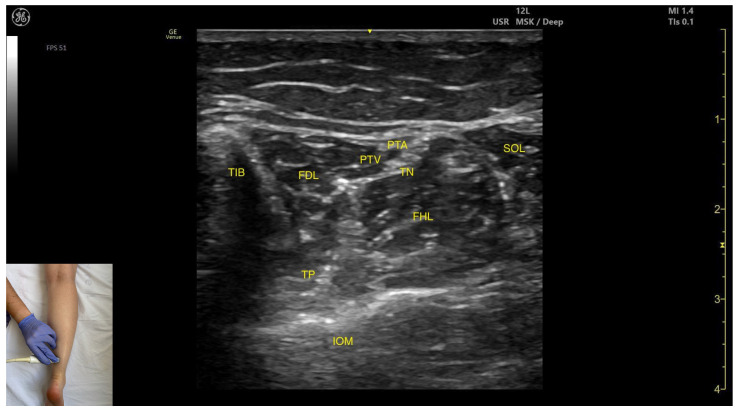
Ultrasound anatomy of the flexor digitorum longus: TIB—tibia; FDL—flexor digitorum longus; TP—tibialis posterior; IOM—interosseous membrane; PTA—posterior tibial artery; PTV—posterior tibial nerve; FHL—flexor hallucis longus; SOL—soleus; TN—tibial nerve.
